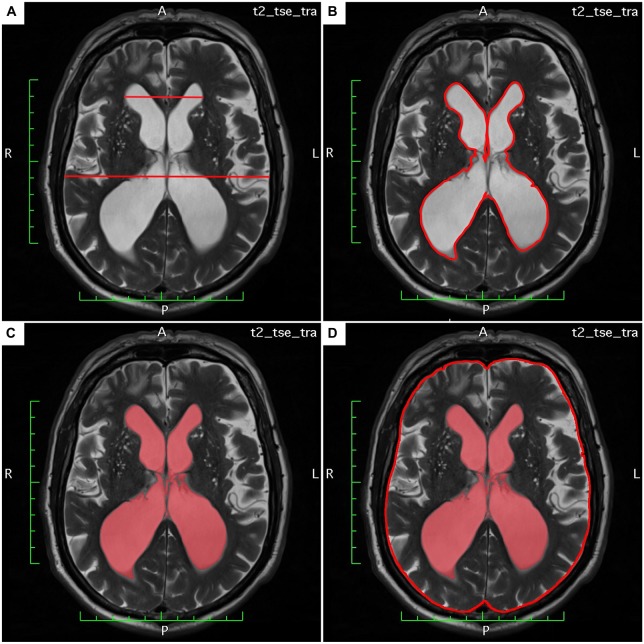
(A–D) Demonstrates estimation of Evan’s Index and lateral ventricles area index (LVAI) on axial T2 weighted (T2w) magnetic resonance imaging (MRI) sections.
